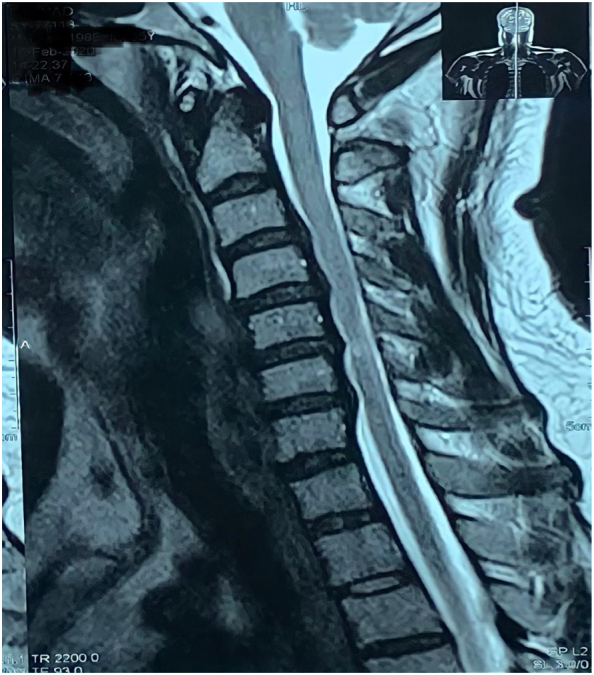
MRI of cervical spine in sagittal T2 image showing multilevel compression at C4 to C7.
